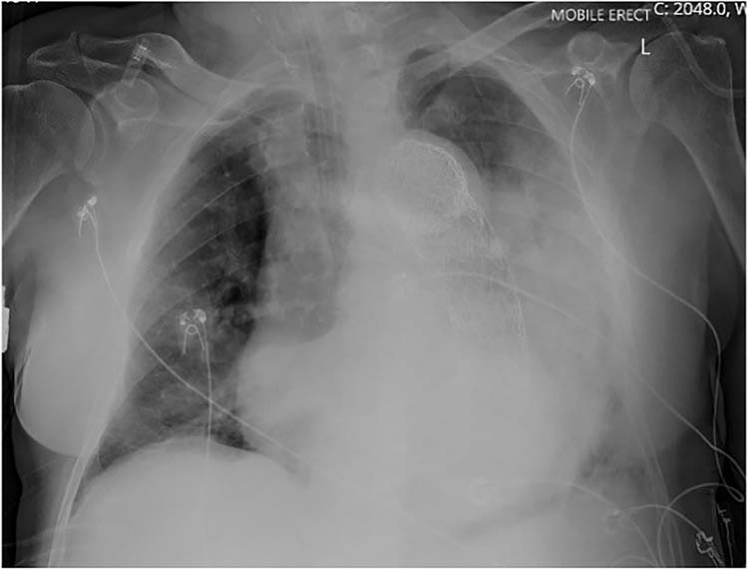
Erect AP chest X-ray indicating opacification of the left mid and lower zones, with thoracic vascular graft *in situ*.
